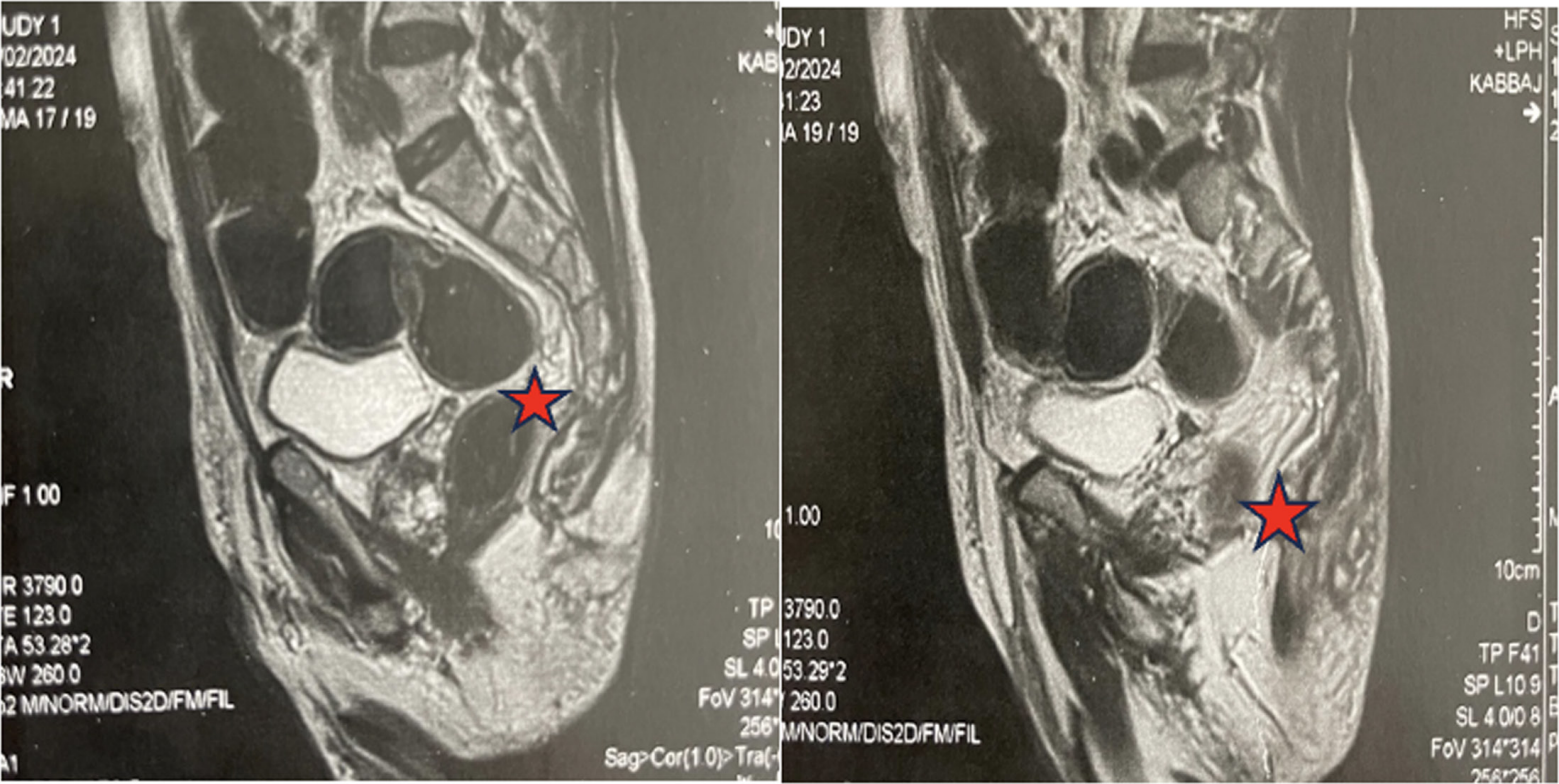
Pelvic MRI shows a pericoccygeal collection with soft tissue involvement and a fistulous tract.
